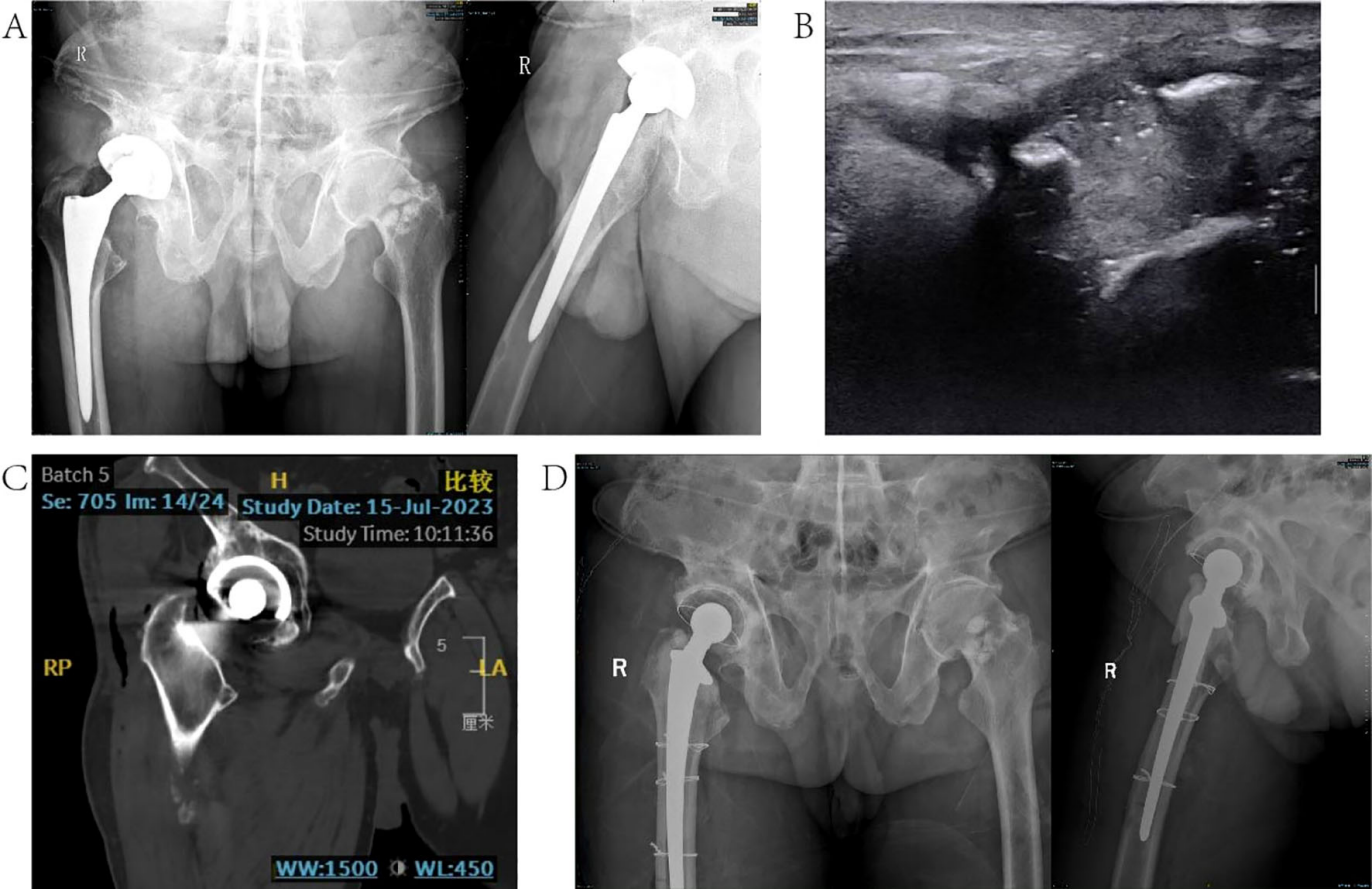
Typical case 2. (A) Frontal and lateral radiographs of the hip joint before the last open surgery; (B) localized ultrasound of the hip joint; (C) CT of the hip joint; (D) frontal and lateral radiographs of the hip joint after the open surgery.
